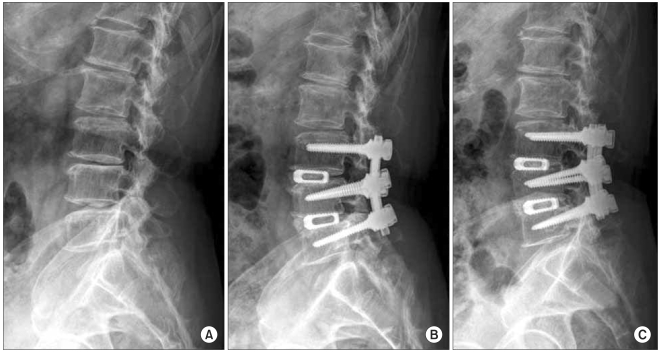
A 71 year-old male who presented with lower back pain and motor weakness. (A) The lateral radiograph shows disc space narrowing on L3-4-5 and degenerative kyphosis. (B) The posterior lumbar interbody fusion with a single cage and a transpedicular instrument was performed and the follow-up radiograph shows maintenance of the disc height and restoration of lordosis. (C) At 3 years after surgery, the lateral radiograph shows solid fusion and maintainance of the reduction.
